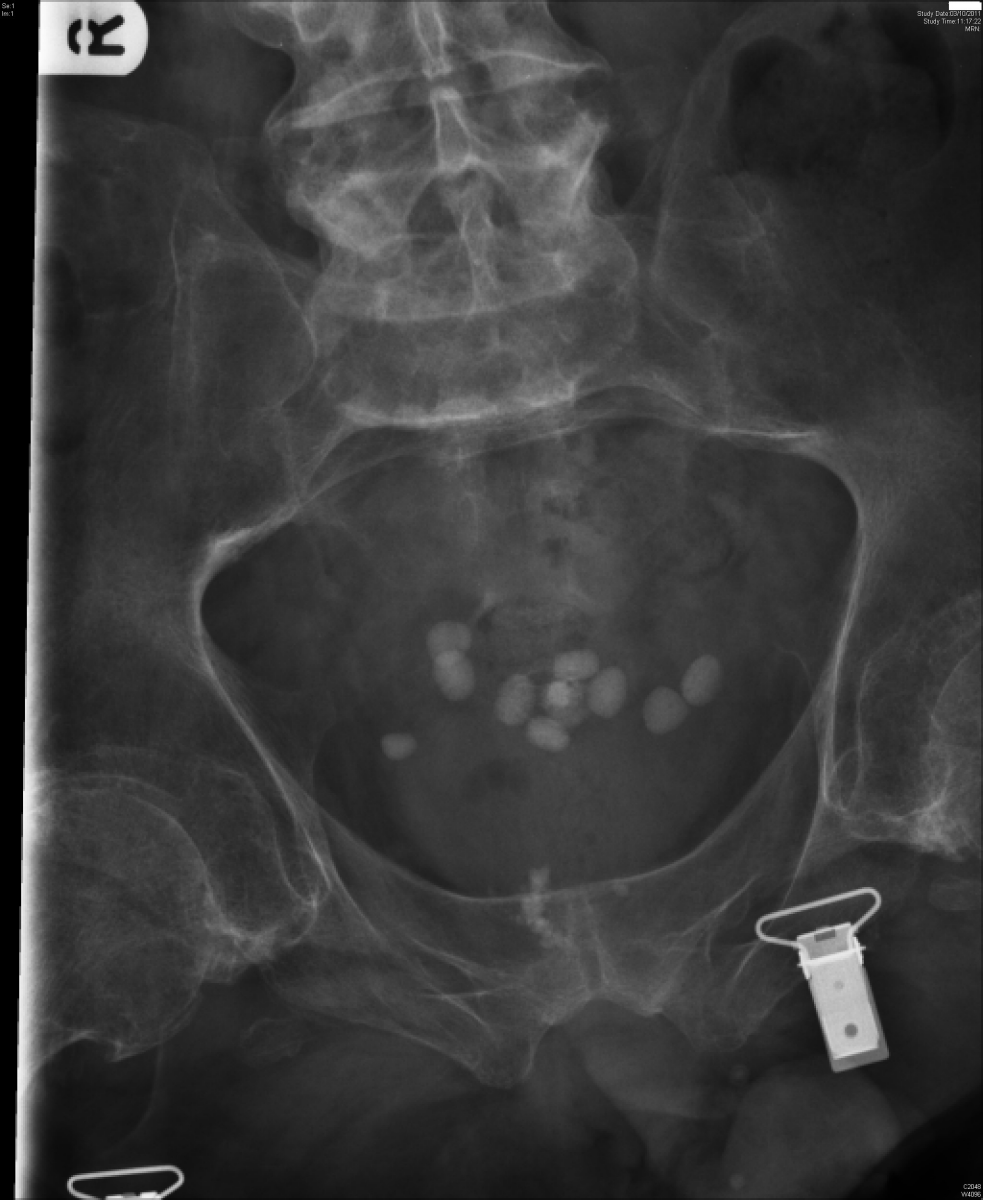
Case number: 2. X-ray of urinary bladder, taken on 03 October 2011, showed multiple calculi within the bladder. This patient developed severe autonomic dysreflexia when lithotripsy was carried out without anaesthesia.
